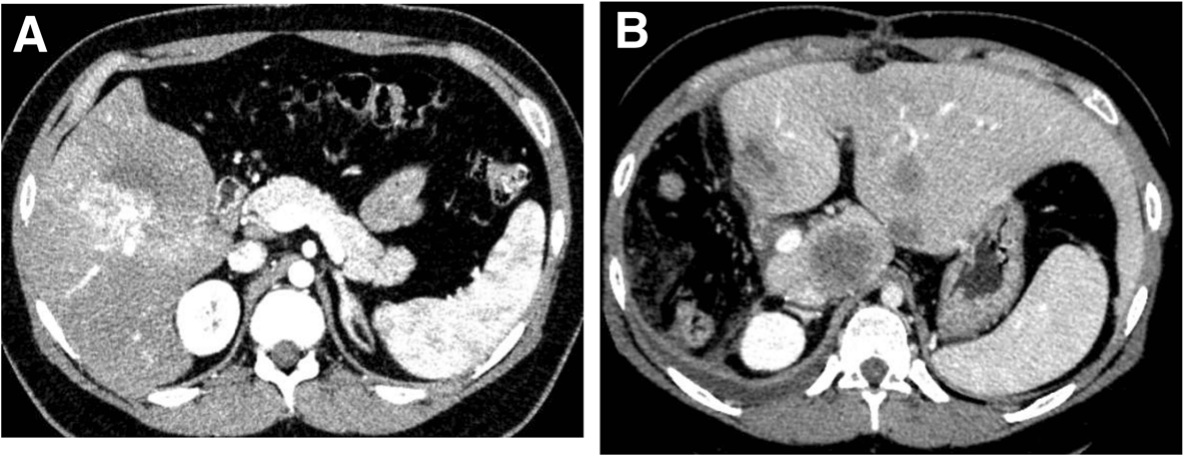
CT findings. a A large, heterogeneously enhanced mass with focal calcification was present in the right lobe of the liver. b Multiple recurrent lesions in the remnant liver and an enlarged para-aortic lymph node on postoperative day 62
